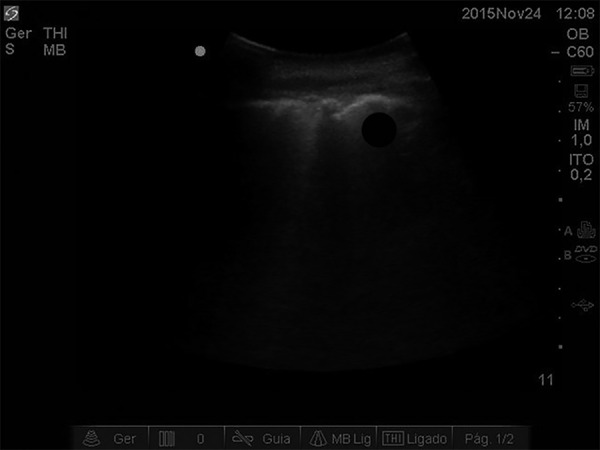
Ultrasound images of the patient's lung, revealing B lines.
